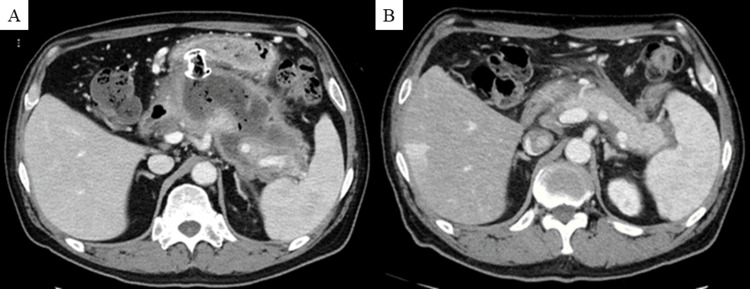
Changes in abdominal CT scans after initiation of treatment for WON (A) Endoscopic ultrasound-guided transgastric drainage using a lumen-apposing metal stent did not improve encapsulated collection significantly (day 98). (B) A follow-up CT after endoscopic necrosectomy shows marked improvement in the encapsulation collection (day 195) CT: computed tomography, WON: walled-off necrosis
